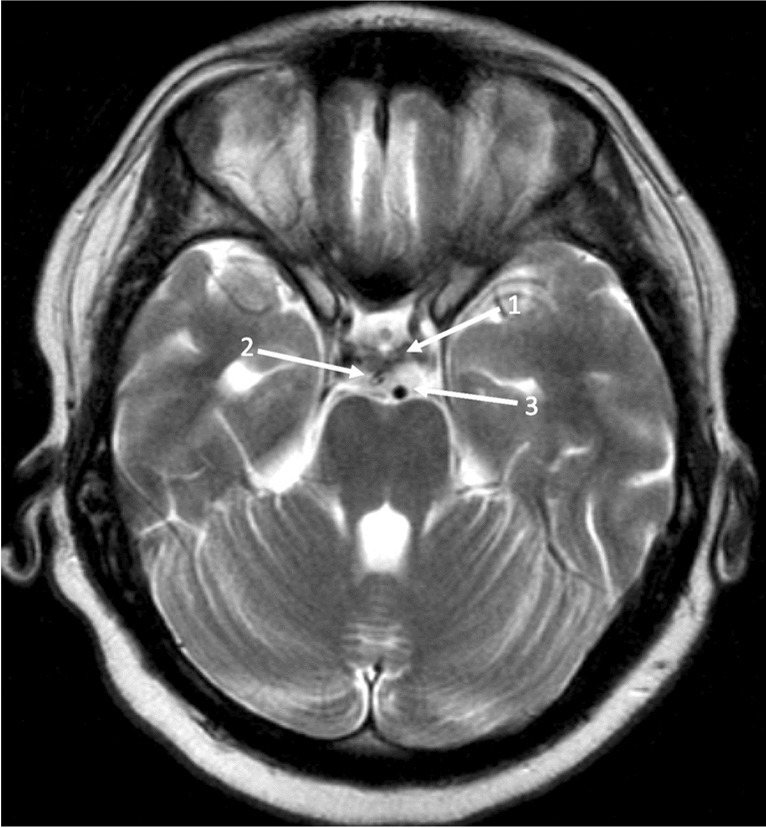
Postoperative MRI of a patient treated with sTVIP. T2-weighted axial MRI showing the cisternal part of the internal shunt (2) posterior to the dorsum sellae (1) and lateral to the basilar artery (3)
